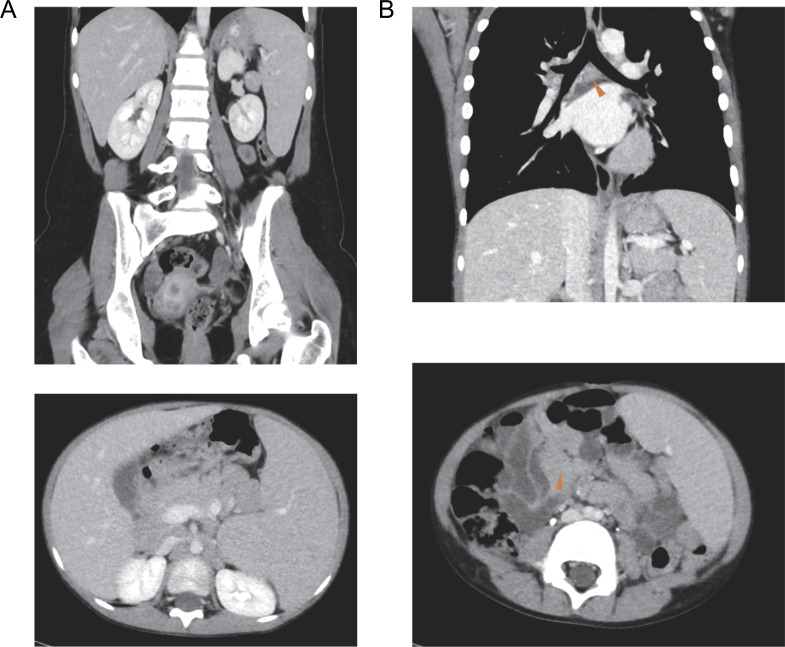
Abdominal enhanced CT. (A) Representative images of splenomegaly. Upper and lower panels depict patients aged 14 and 6 years, respectively. (B) Representative images of lymphoproliferation, with lesions of interest indicated by orange arrows. Upper and lower panels depict patients aged 10 and 6 years, respectively.
